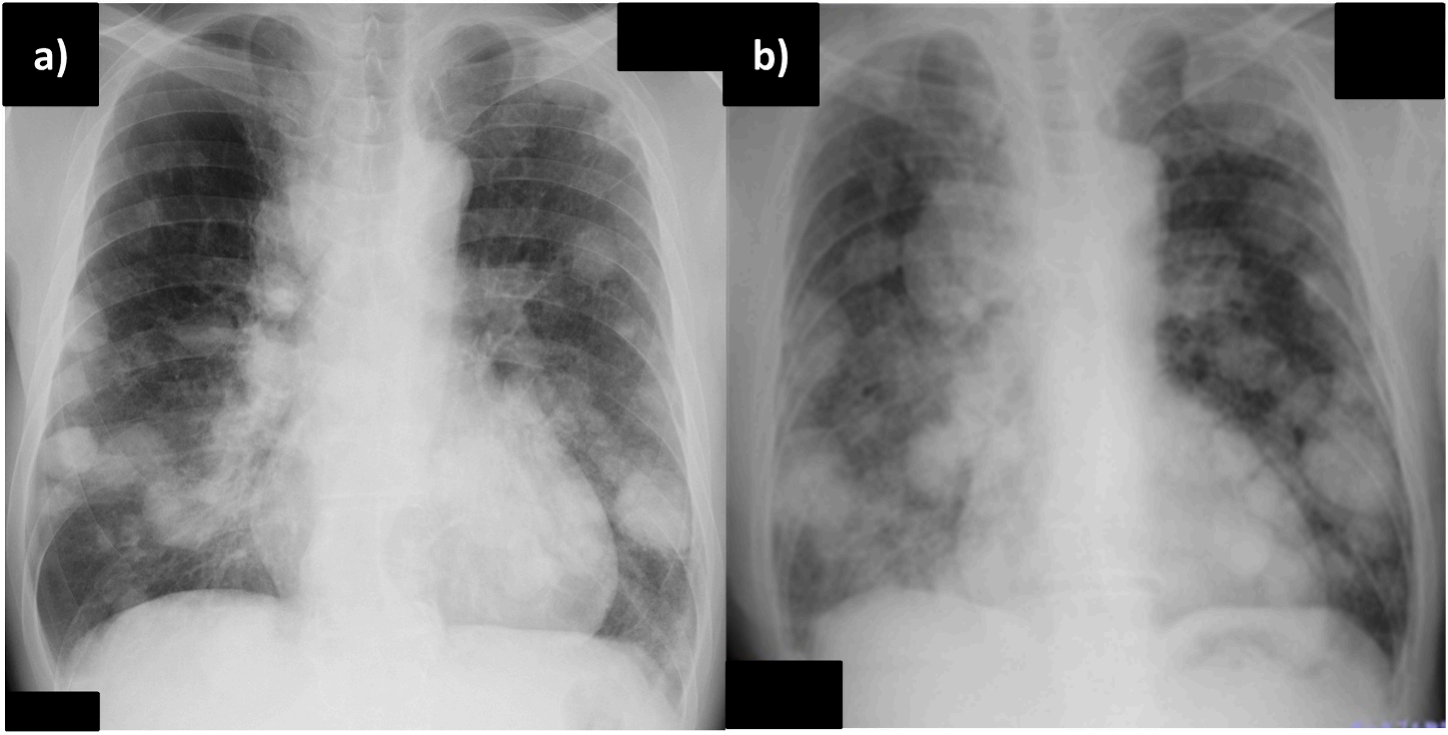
Chest radiograph showing multiple nodules and tumors in the bilateral lung fields a) on admission and b) on the 20th day of hospitalization.
